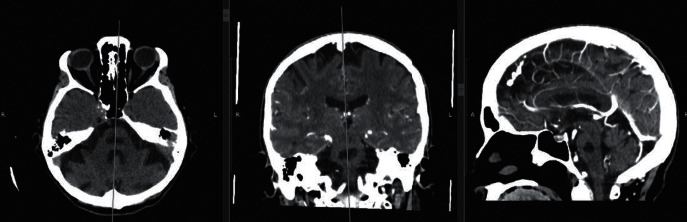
Cranial computed tomography showing a hypoattenuating focal area in the central region of the pons (sequences: axial, coronal, and sagittal).
